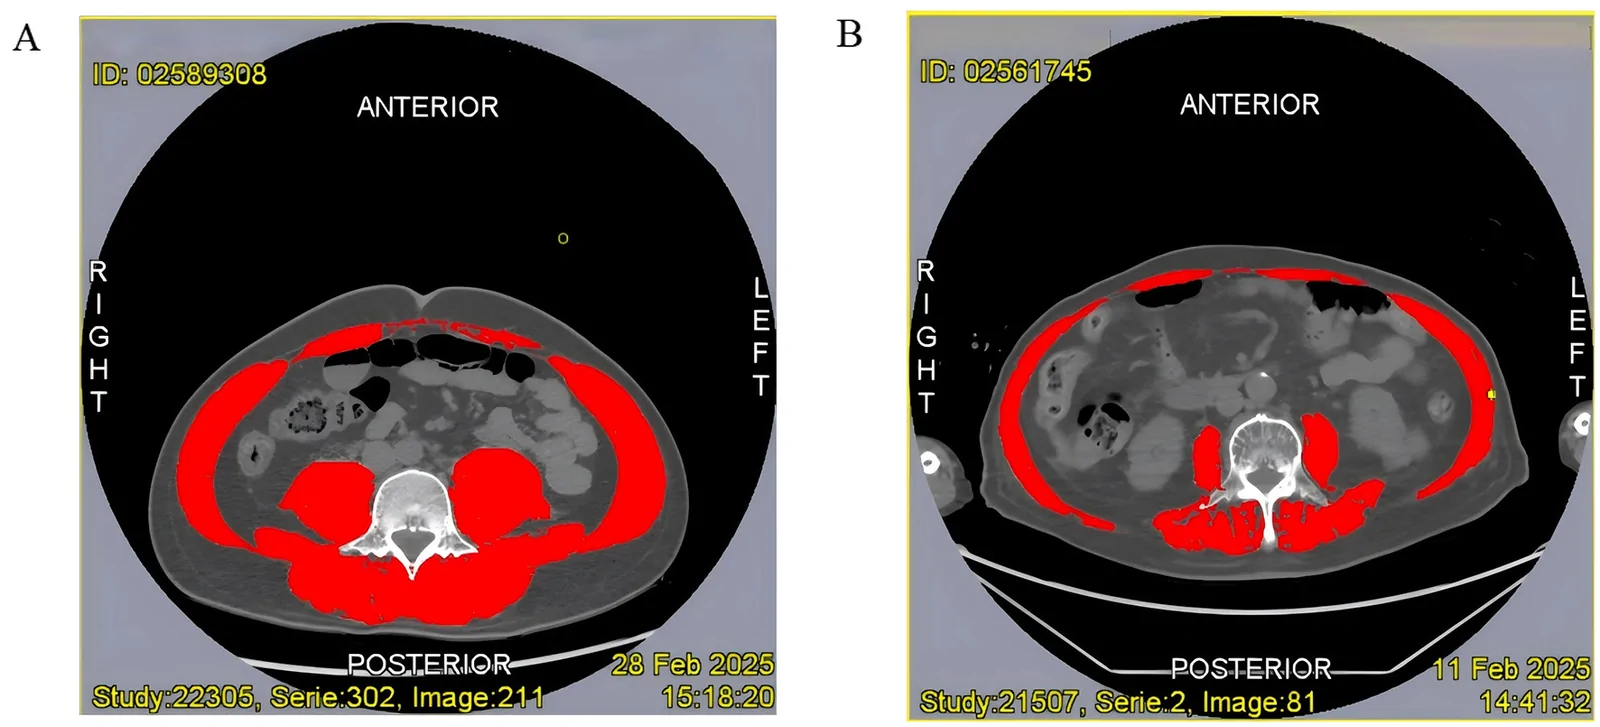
Skeletal muscle area on L3 axial slice. Measuring skeletal muscle area on L3 axial slice Computer-Tomography (CT) Image was measured by the SliceOmatic software (5.0 Rev-9). Radio density thresholds were selected to correlate with those of skeletal muscle, with lower and upper thresholds of −29 and 150 Hounsfield units, respectively. (A) normal muscle mass; (B) low muscle mass.
